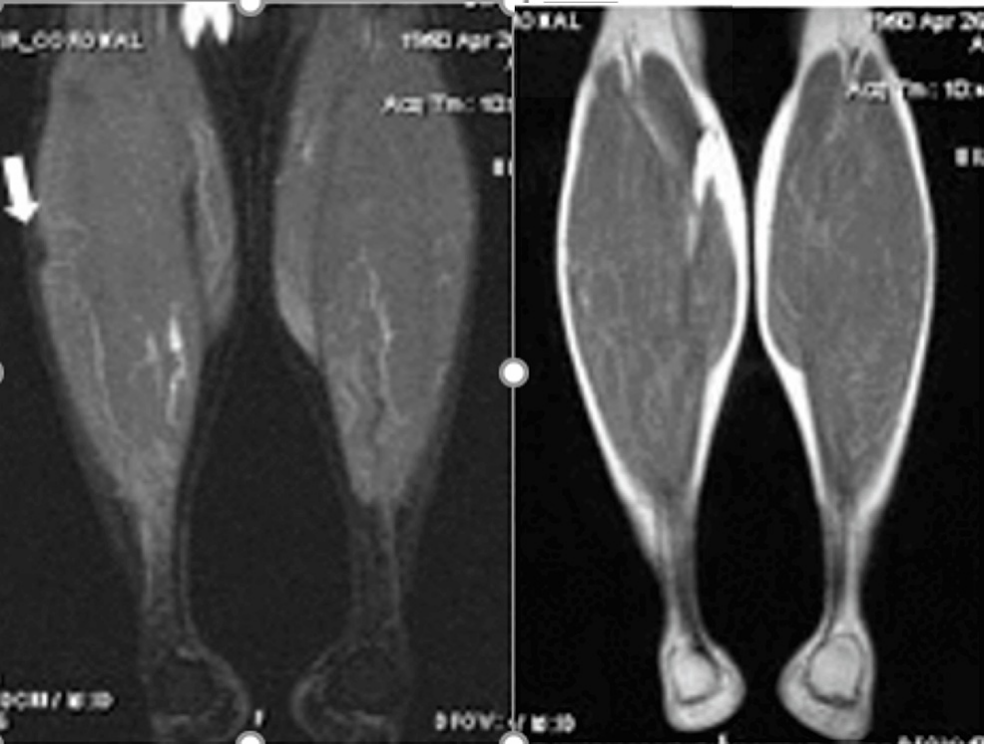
Magnetic resonance imaging (MRI) of both calves after 15 months of therapy Coronal T2 short-tau inversion recovery (STIR) image of both calves, on the left, demonstrating resolution of abnormal increased signal and subtle residual low signal. Corresponding coronal T1-weighted images, on the right, do not demonstrate any signal abnormality.
